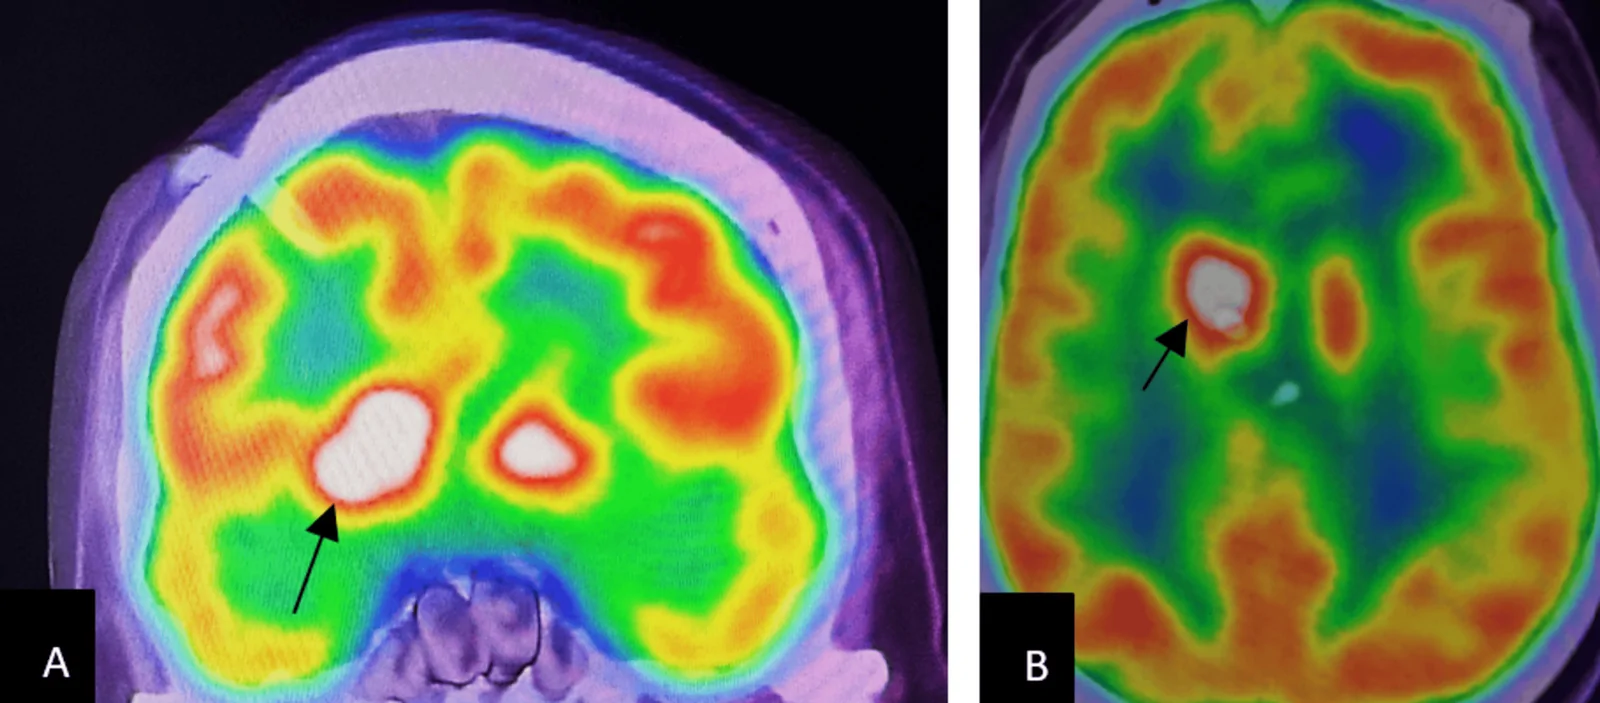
PET findings (A) PET coronal FDG-PET scan demonstrating marked SVUmax 10.5 hypermetabolism in the periventricular regions and basal ganglia (black arrow). (B) Axial FDG-PET hypermetabolism in the periventricular regions (black arrow) FDG-PET: fluorodeoxyglucose positron emission tomography
